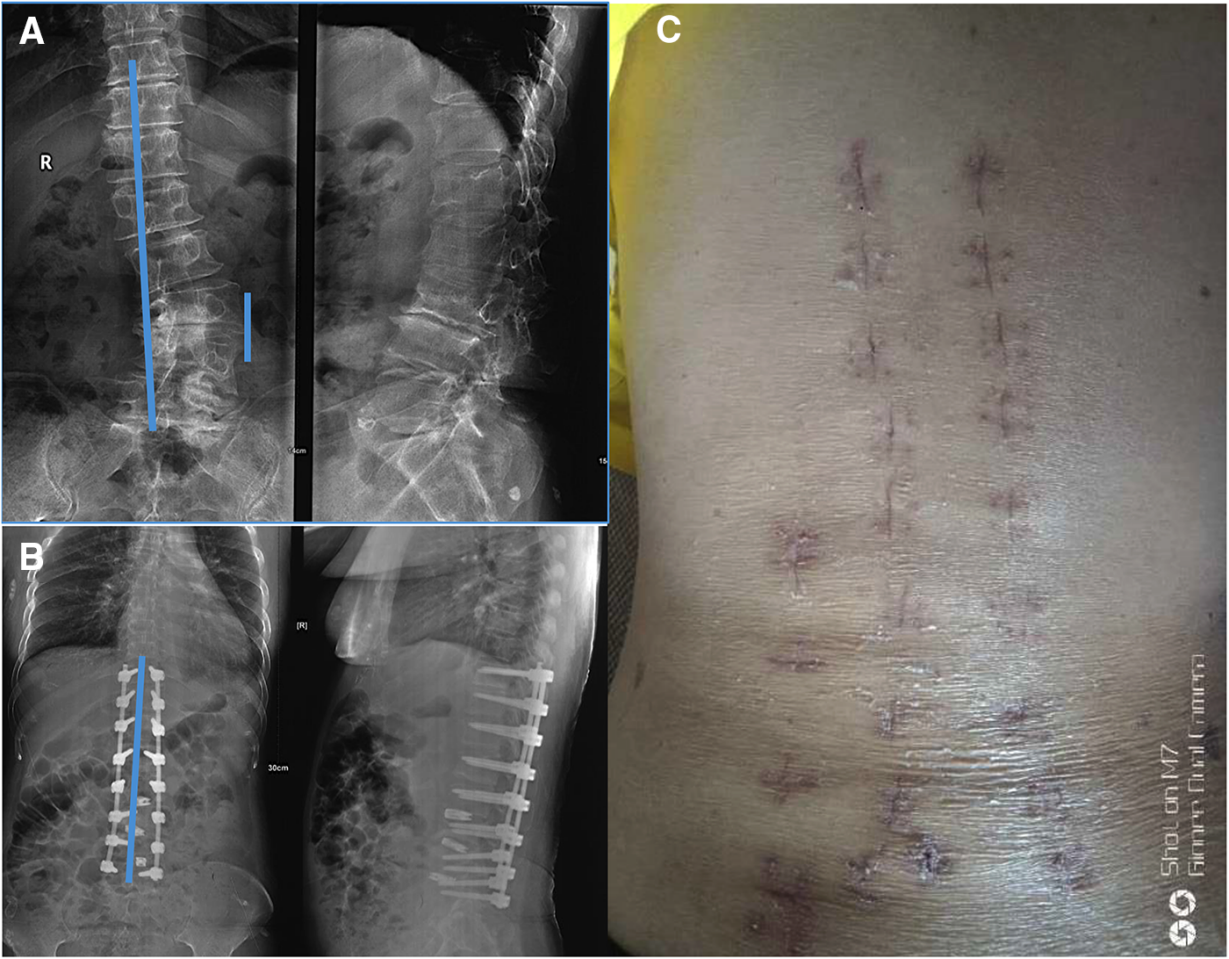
X-rays of the 56-year-old female patient with ADS, which was taken on November 2018: (A) before operation, (B) after operation, and (C) the patient's postoperative appearance.
